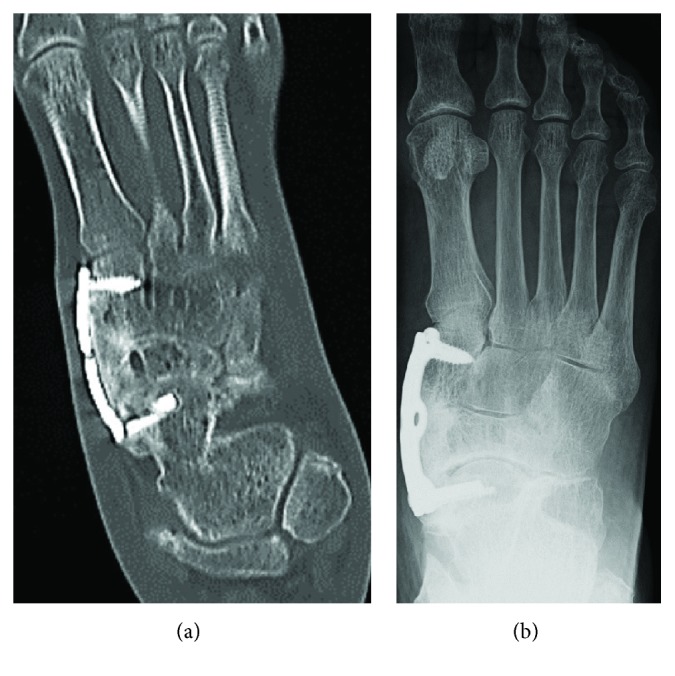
(a) CT scan 6 months after surgery shows sufficient bone union fusion at the talonavicular and naviculocuneiform joints. (b) After 5 years of follow-up, the radiograph shows sufficient fusion.
